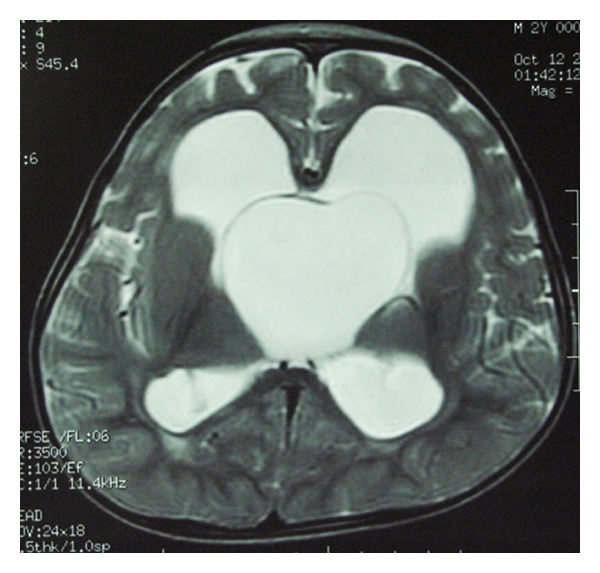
The preoperative of MRI image showed a cyst lesion at the suprasellar cistern which compressed the third ventricle (T2 weighted image).
